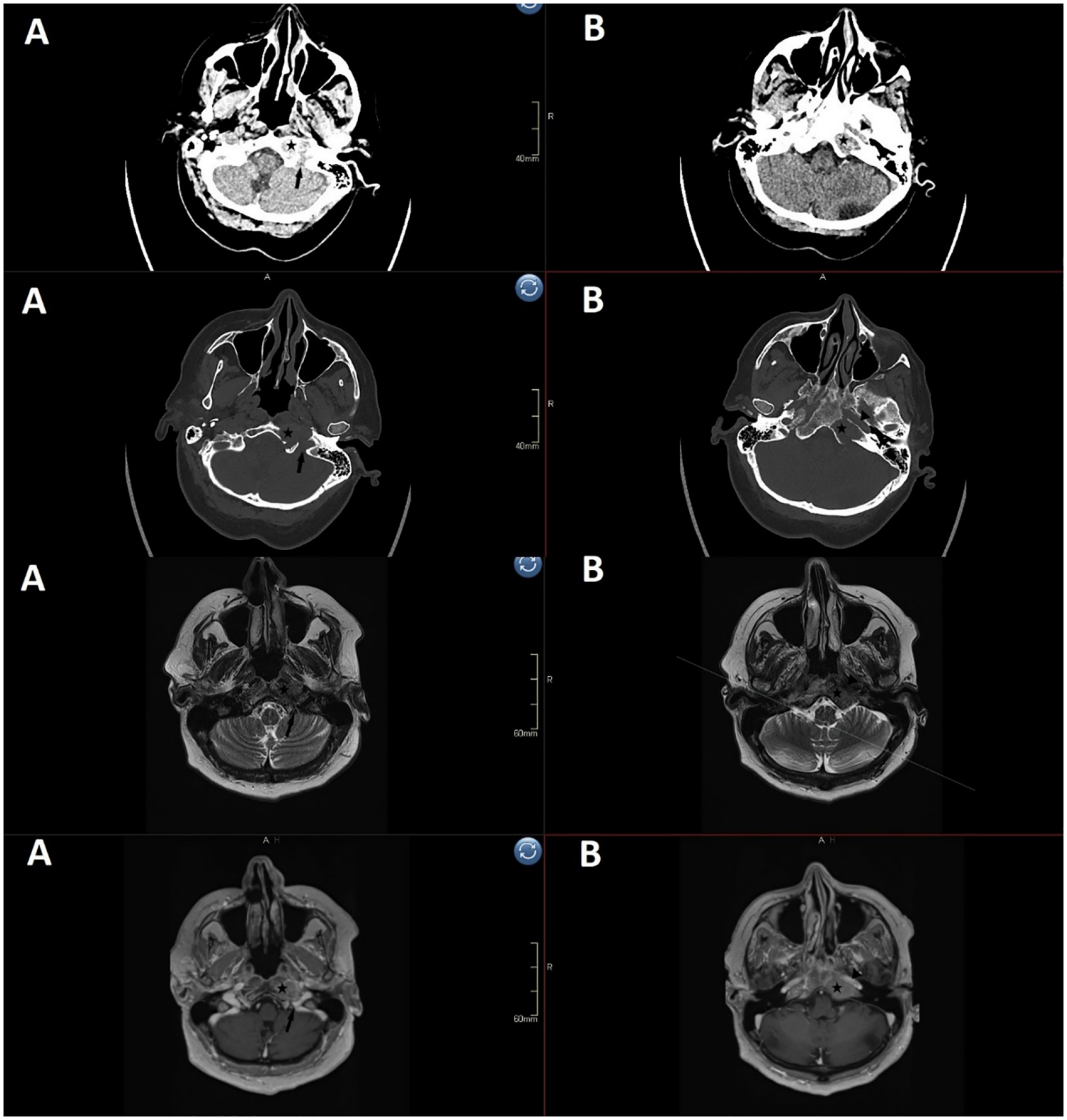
Preoperative imaging (1st row axial CT soft tissue window, 2nd row axial CT bone window, 3rd row axial T2 sequence, 4th row axial contrast-enhanced T1 sequence) shows a 2.4 x 2 x 1.5 cm erosion in the clivus and left petrous apex. The left condyle is intact, but the tumor invades the hypoglossal canal and extends to the jugular foramen (A), erodes most of the lateral clivus from the petrosal part of carotid artery to the jugular tubercle (B), and extends posterior to the paraclival carotid and petroclival fissure to via medial petrous apex toward the internal auditory canal. *(star: tumor, arrow: jugular foramen, triangle: petrosal part of carotid)*.
